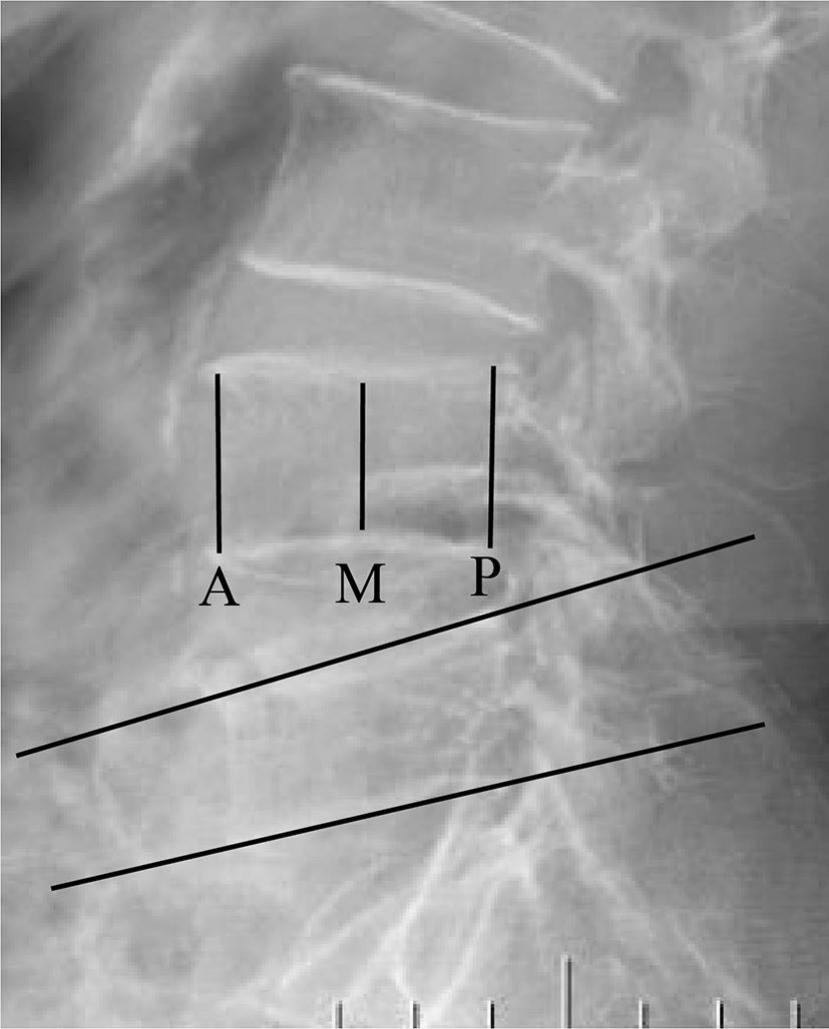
The local Cobb angle was measured to determine the kyphotic deformity on lateral radiographs. A, M and P represented anterior, middle and posterior height of vertebral body, respectively. The height of the fractured vertebral body was assessed via the compression ratio before and after operation, the compression ratio was computed by A/P $$\times$$ 100% and M/P $$\times$$ 100%.
